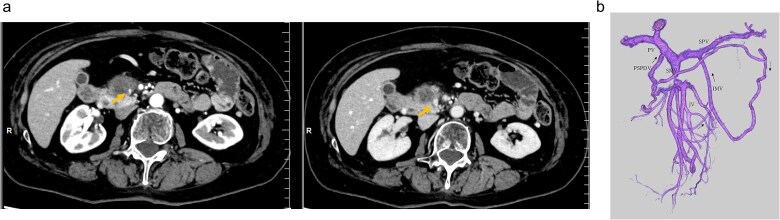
(a) Preoperative contrast-enhanced CT in the portal venous phase demonstrated a hypovascular tumor measuring 20 mm in the pancreatic uncinate process and mild improvement in SMV narrowing (arrow, pre-NAC in left image, post-NAC in right image). (b) Preoperative 3D imaging demonstrated that the jejunal veins were discontinued separately, and there are three collateral pathways, via posterior superior pancreaticoduodenal vein, inferior mesenteric vein through the right gastroepiploic vein, and stenotic SMV with intramesenteric anastomoses. SMV: Superior mesenteric vein, NAC: Neoadjuvant chemotherapy.
